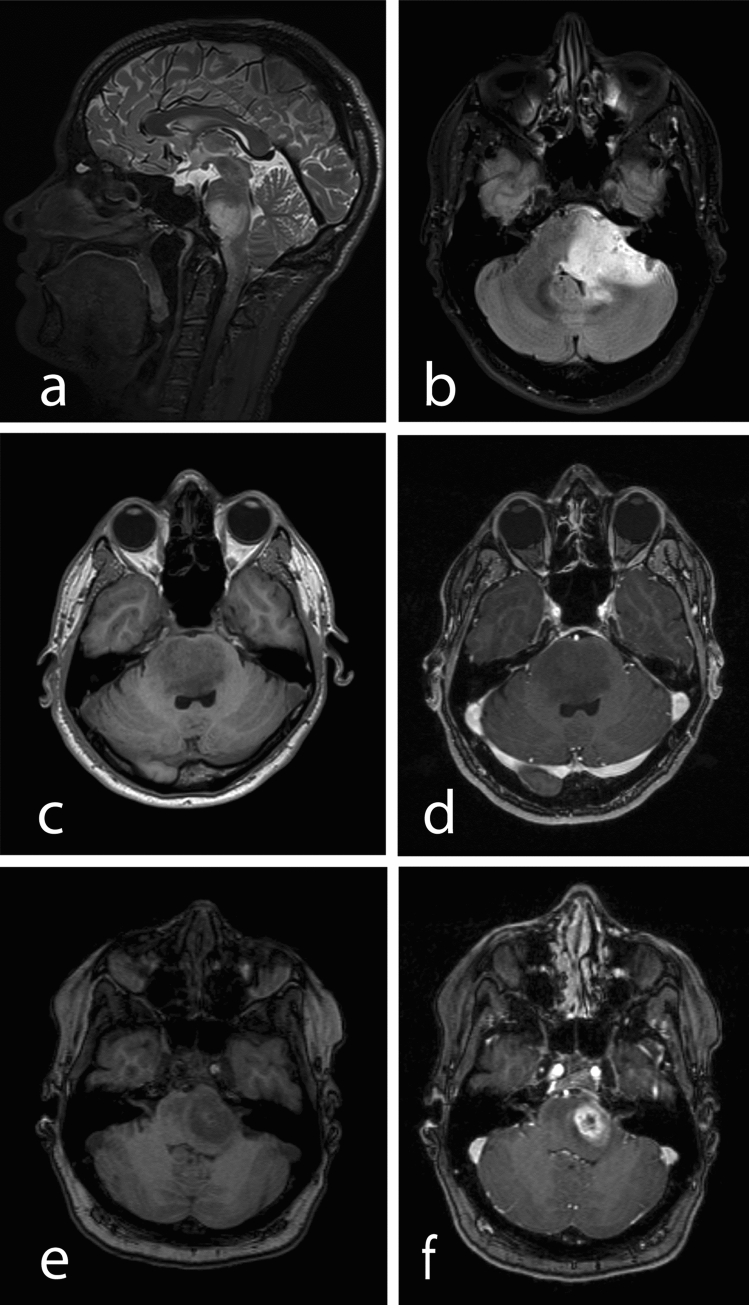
Sagittal T2-weighted MR image representing a brain stem glioma located in the pons and medulla oblongata (a) Axial FLAIR image depicting a brain steam glioma located in the pons, the left middle cerebellar peduncle and the left cerebellar hemisphere (b) brain stem glioma located in the pons without contrast enhancement on T1-weighted MR images before (c) and after (d) contrast media application. Brain stem glioma located in the pons with markedly contrast enhancement and central necrosis on T1-weighted MR images before (e) and after (f) contrast media application
